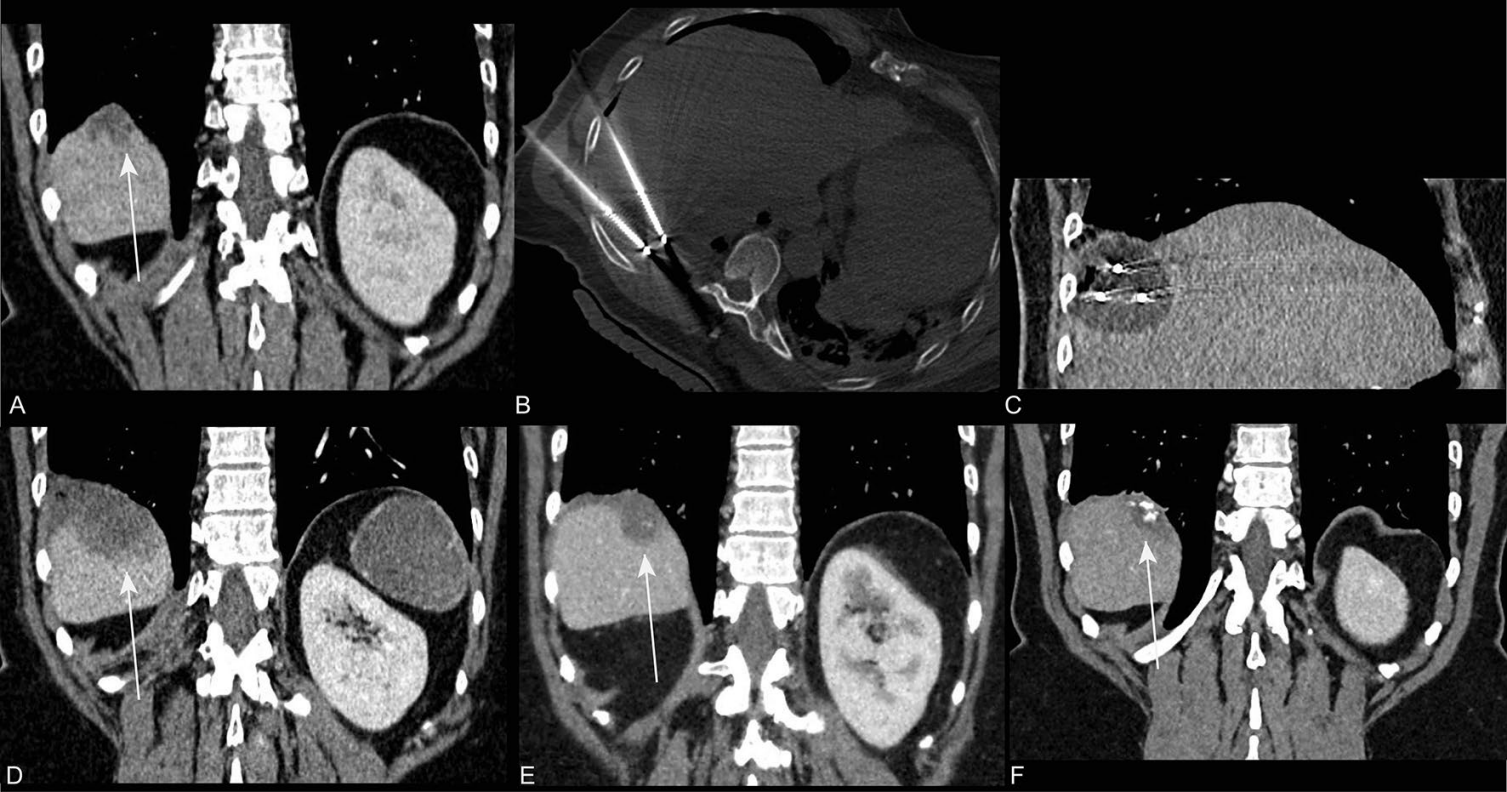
A 57-year-old female patient with a history of mucinous appendix carcinoma treated with a right hemicolectomy, two debulkings including HIPEC, and a liver metastectomy, now presents with a rise in carcinoembryonic antigen (CEA) from 8.0 to 38 µg/L. CT shows a peritoneal metastases of 32 mm located on the diaphragm invading the liver (A). Cryoablation was performed using three needles (B; transverse CT, C; sagittal CT). Coronal CT images show complete ablation after one month (D), and local control after 5 months (E) and 3 years (F) with a stable CEA varying between 5.6 and 7.0 µg/L
